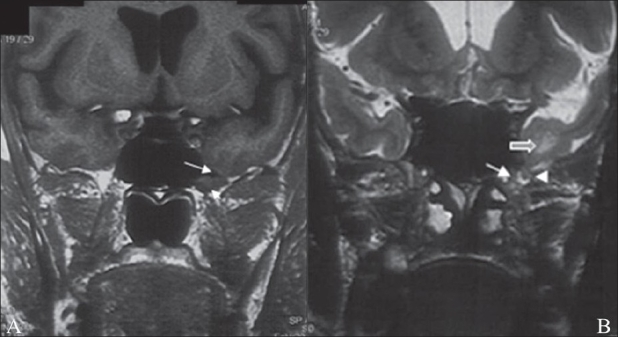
Coronal T1W MRI image (A) shows a small defect in the left inferolateral wall of the sphenoid sinus (large arrow) through which a thin tongue of anteromedial left temporal lobe (small arrow) is seen herniating into the sinus cavity. Coronal T2W image (B) shows a small cerebrospinal fluid-filled meningeal sac (arrow) herniating through the bony and dural defect (arrowhead) in the left anterolateral wall of the sphenoid sinus. This meningeal sac contains a tongue of the anteromedial temporal lobe. Focal hyperintensity is seen involving the white matter of the anteromedial temporal lobe (block arrow), which represents focal gliosis
